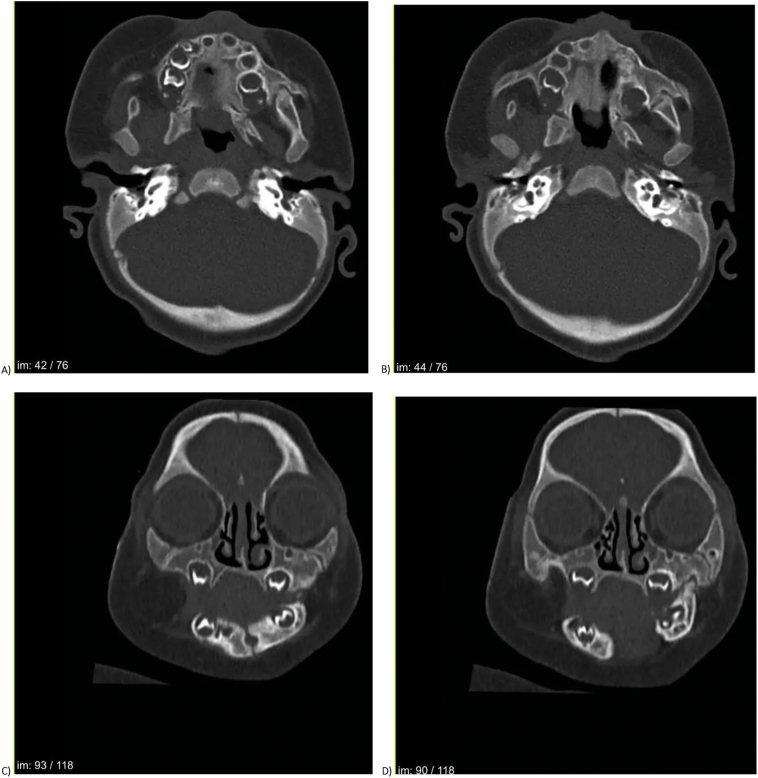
(A, B) Axial CT scan demonstrating bony fusion between the maxilla and mandible. (C, D) Coronal CT scan showing the extent of fusion at the alveolar level without involvement of the ramus or zygomatic arch.
